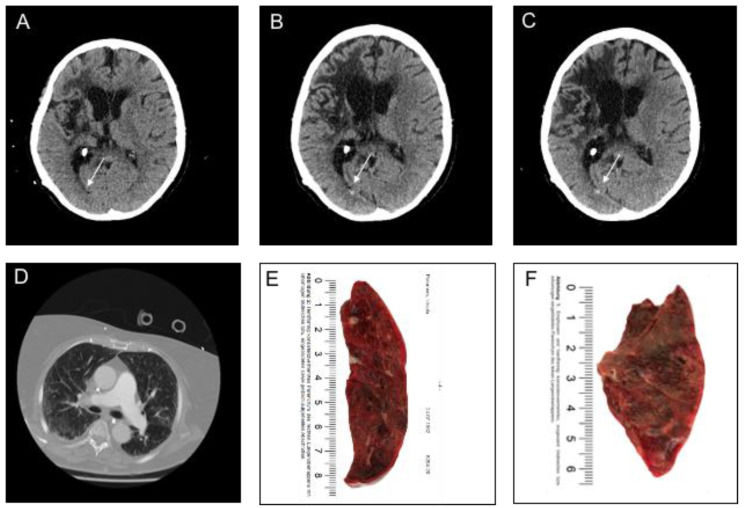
Representative computed tomography (CT) images of a patient with Coronavirus Disease 2019 with an intraventricular hemorrhage (IVH). Legend: (A,D): Brain CT image (A) with corresponding chest CT image at admission (D). (B,C): Brain CT image eight days (B) and ten days after hospitalization (C). (F): Longitudinal section of the right lung indicative of purulent, hemorrhagic pneumonia in the upper lobe. (E,F): Longitudinal section of the left lung indicative of purulent, hemorrhagic pneumonia and emphysematous structural alterations in the upper lobe. White arrow indicates acute intraventricular hemorrhage.
